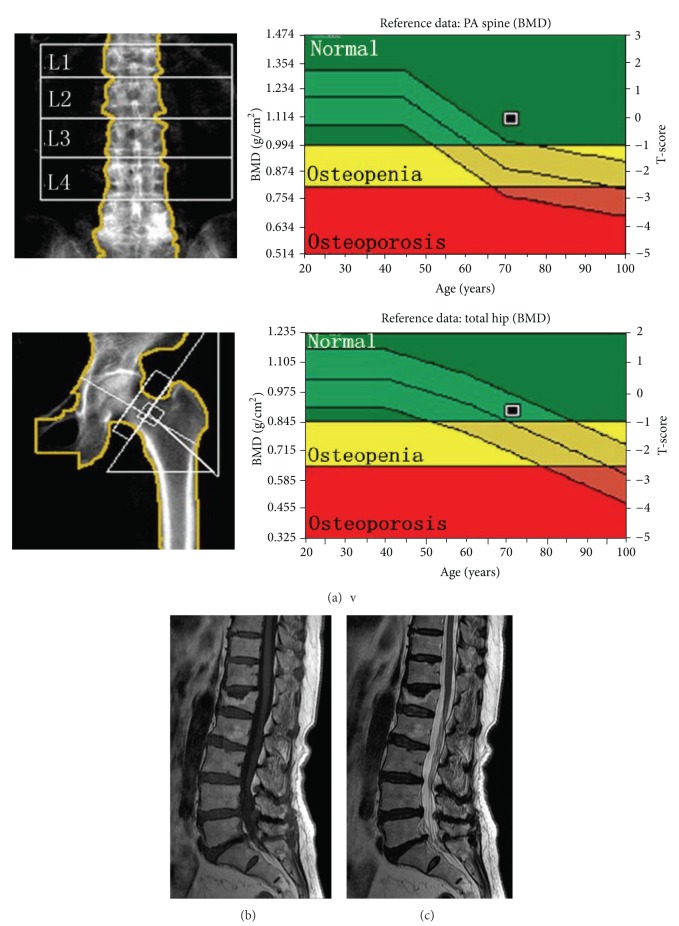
Images obtained from a 71.7-year-old woman whose bone mineral density was found to be normal on dual X-ray absorptiometry. (a) The T-scores for lumbar posteroanterior dual X-ray absorptiometry, the femoral neck, and the total hip were −0.1, −0.8, and −0.7, respectively. The trabecular bone mineral density of L2–L4 was 36.1 mg/cm^3^, and the diagnosis via lumbar quantitative computed tomography was osteoporosis. Sagittal lumbar spine images show two adjacent vertebral fractures with wedging, deformation of the end plates, and degenerative disc disease: (b) T_1_ weighted and (c) T_2_ weighted.
